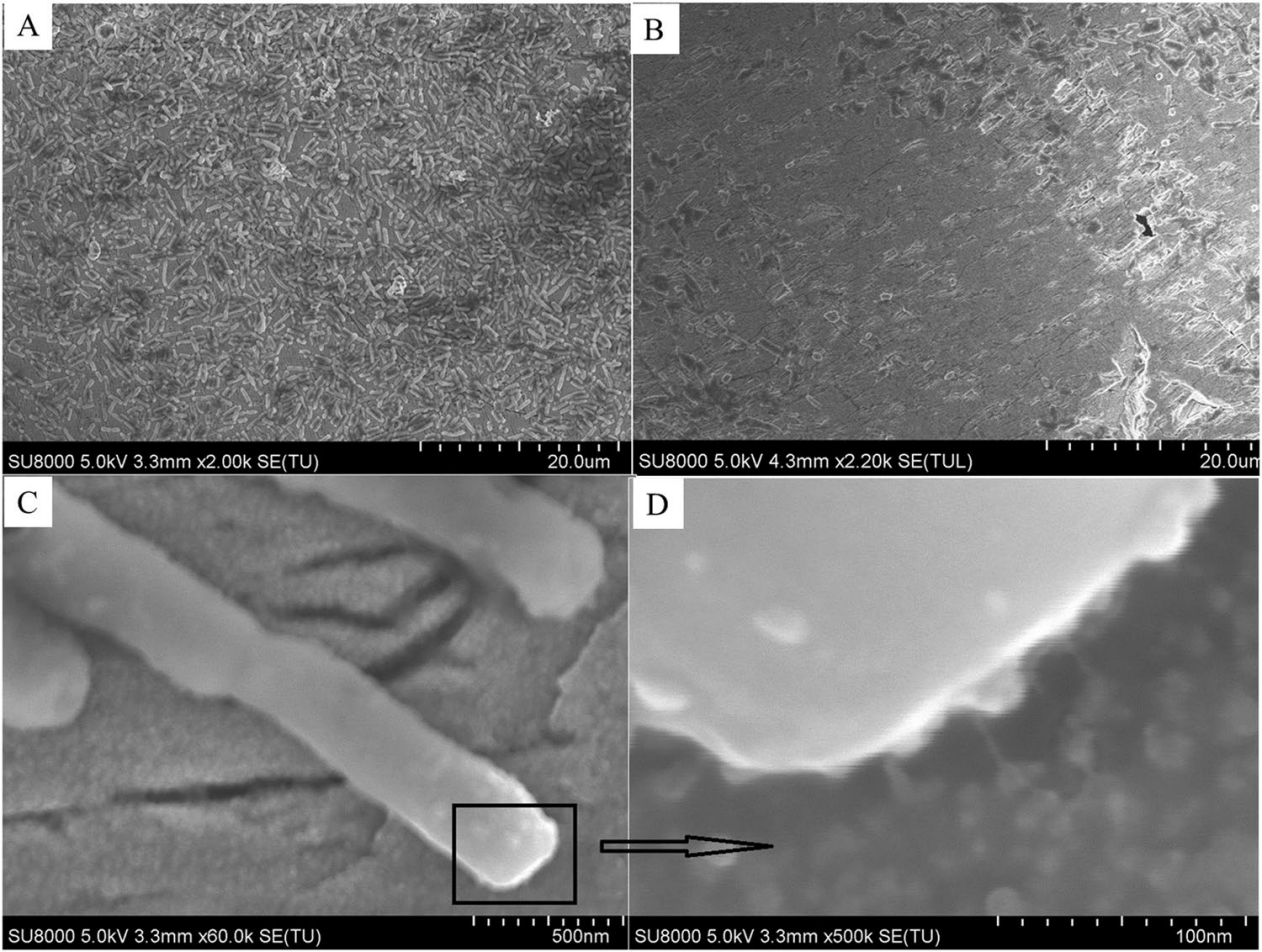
SEM images of pyrite coupons after 47 days leaching with Krouse medium at a pH of 1.55 (A) Macroscopic images of bacteria attached to a pyrite coupon. (B) Image of the pyrite surface after removal of the most strongly attached bacterial cells by ultrasonic treatment with 0.5% (w/v) Tween 80 solution. (C) Image of an individual cell attached to the pyrite surface. (D) Magnified image of the area shown in the square of (C). The smaller arrows in (D) indicate the locations of the pilis and EPS of a single cell. Scale of magnification shown at the bottom of each image.
